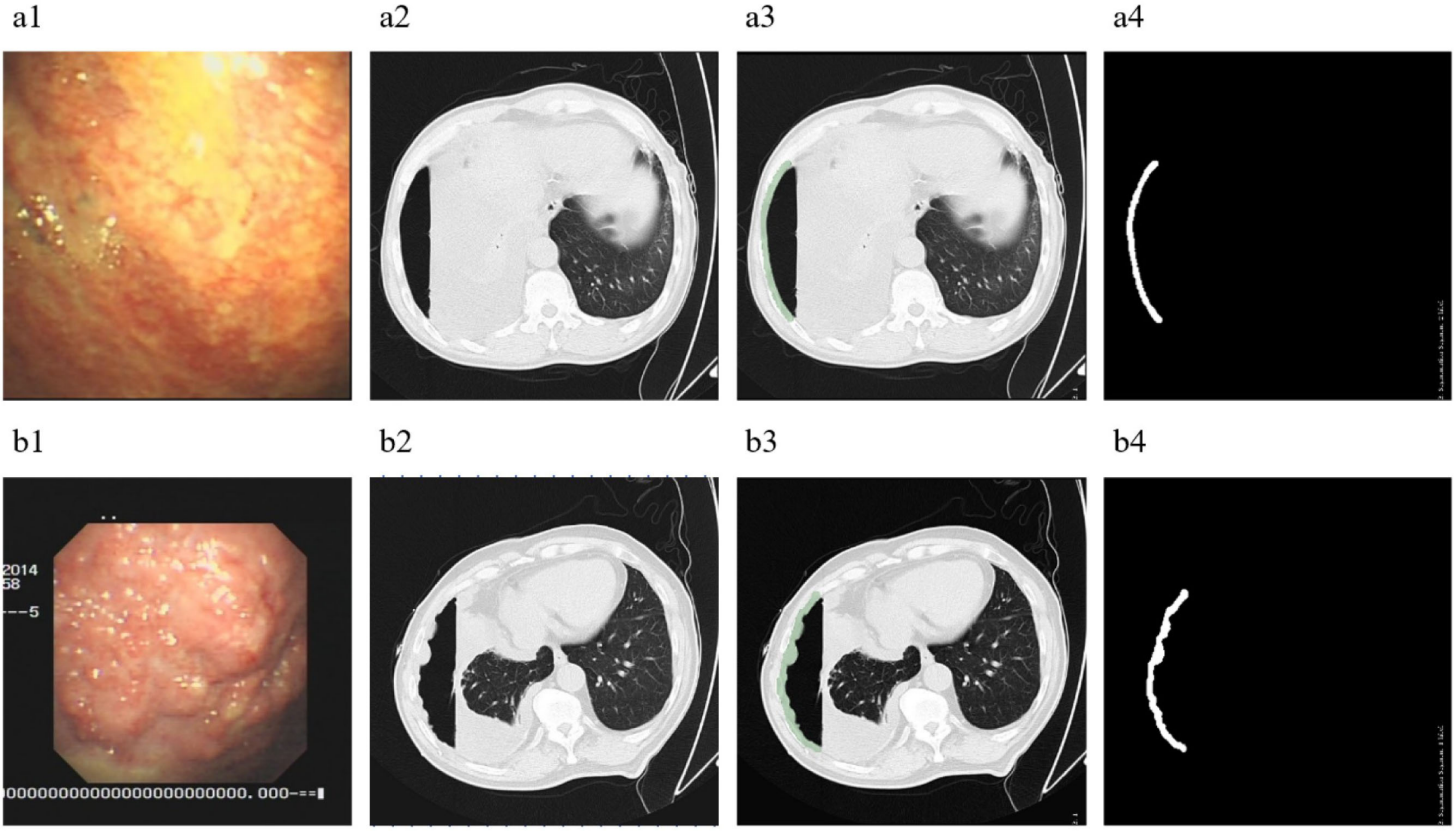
ROI segmentation. (A), A 62-year-old male with PE, diagnosed as tuberculous pleurisy. (B), A 67-year-old male with PE, diagnosed as malignant pleural mesothelioma. 1, Images of pleural lesions under medical thoracoscopy. 2, CT images of patients after artificial pneumothorax. 3, Regions of interest segmented on the CT under lung window. 4, The regions of interest obtained after segmentation.
